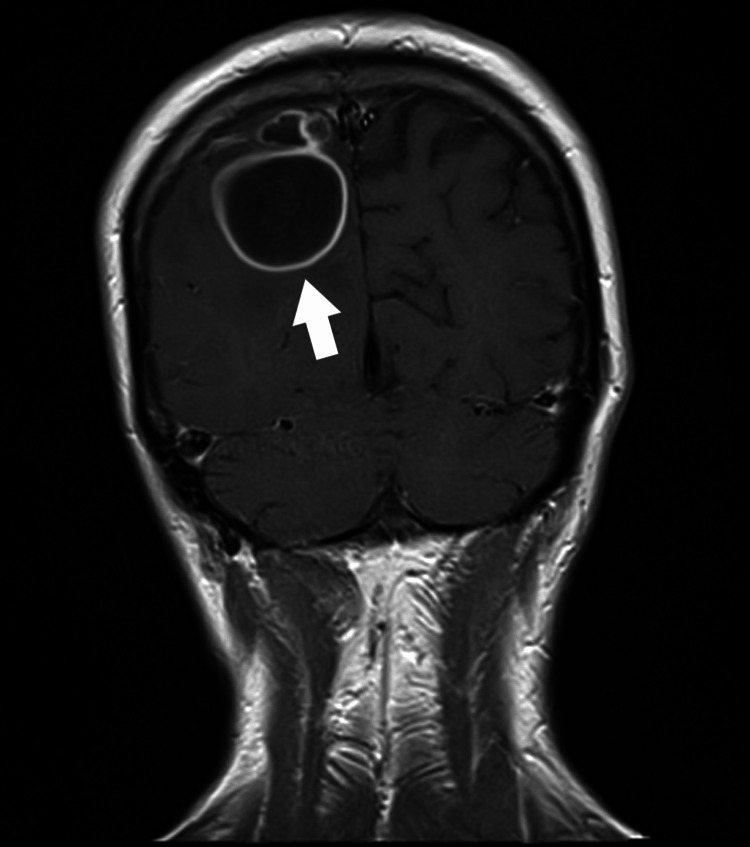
A follow-up MRI scan of the head revealed a residual abscess located in the right parieto-occipital region: anterior-posterior view (marked with a white arrow).
